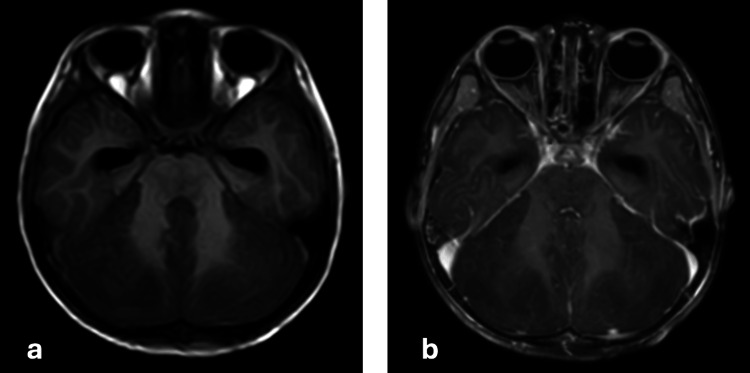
MRI axial T1-weighted (a) plain and (b) contrast images, showing no significant leptomeningeal or parenchymal enhancement MRI: magnetic resonance imaging
